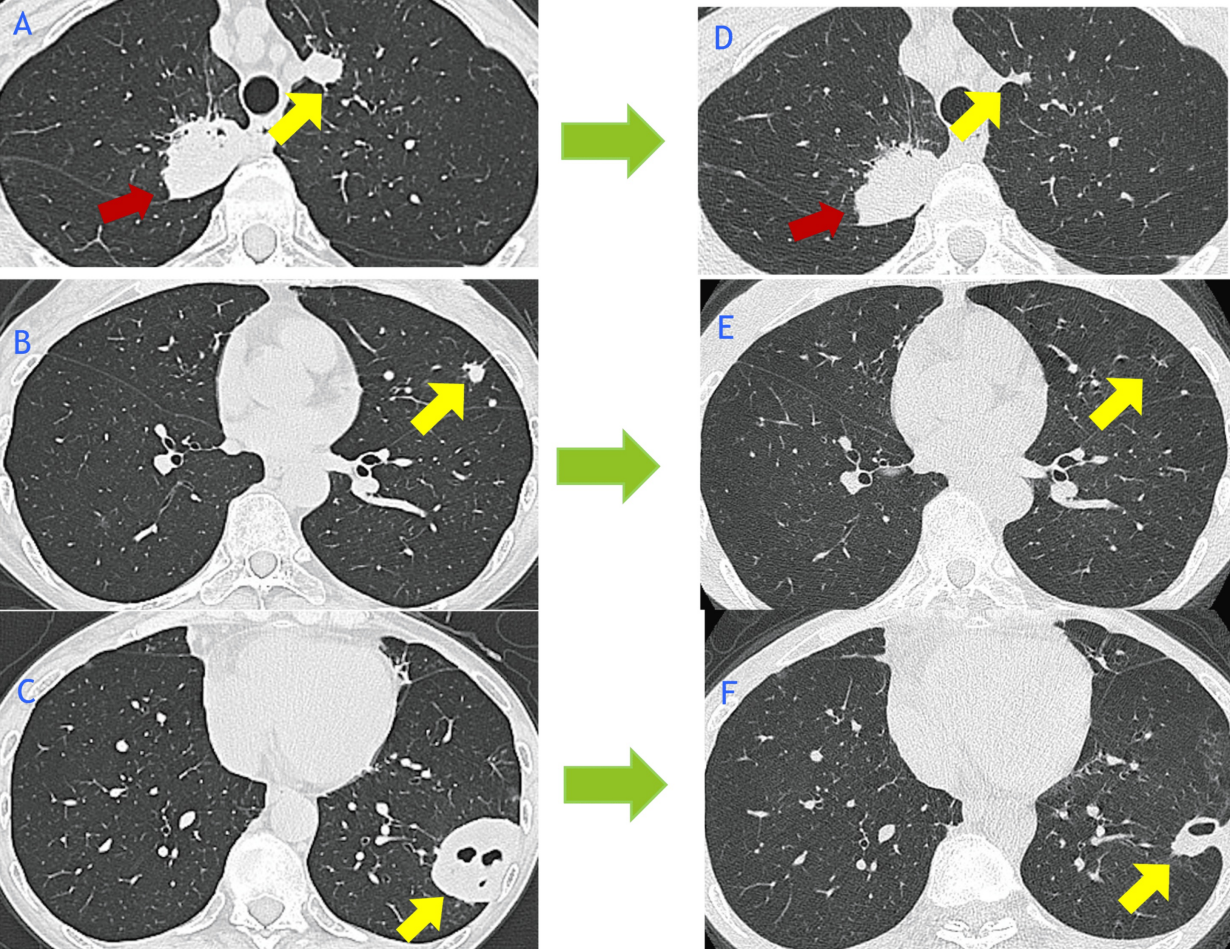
Chest CT findings before and after antifungal therapy for pulmonary cryptococcosis CT images were obtained in X year Y month (A–C) and Y+4 month (D–F). Red arrows indicate the primary lung tumor, which remained stable after treatment. Yellow arrows indicate cryptococcal lesions, which resolved or decreased in size following antifungal therapy. The initial treatment consisted of intravenous liposomal amphotericin B (4 mg/kg/day, 140 mg/day) plus oral flucytosine (100 mg/kg/day, 4000 mg/day in divided doses), administered for 20 days from hospital day 10 to day 30. After CNS involvement was ruled out, the regimen was de-escalated to oral fluconazole (400 mg/day), which was continued thereafter. CT: computed tomography, CNS: central nervous system
